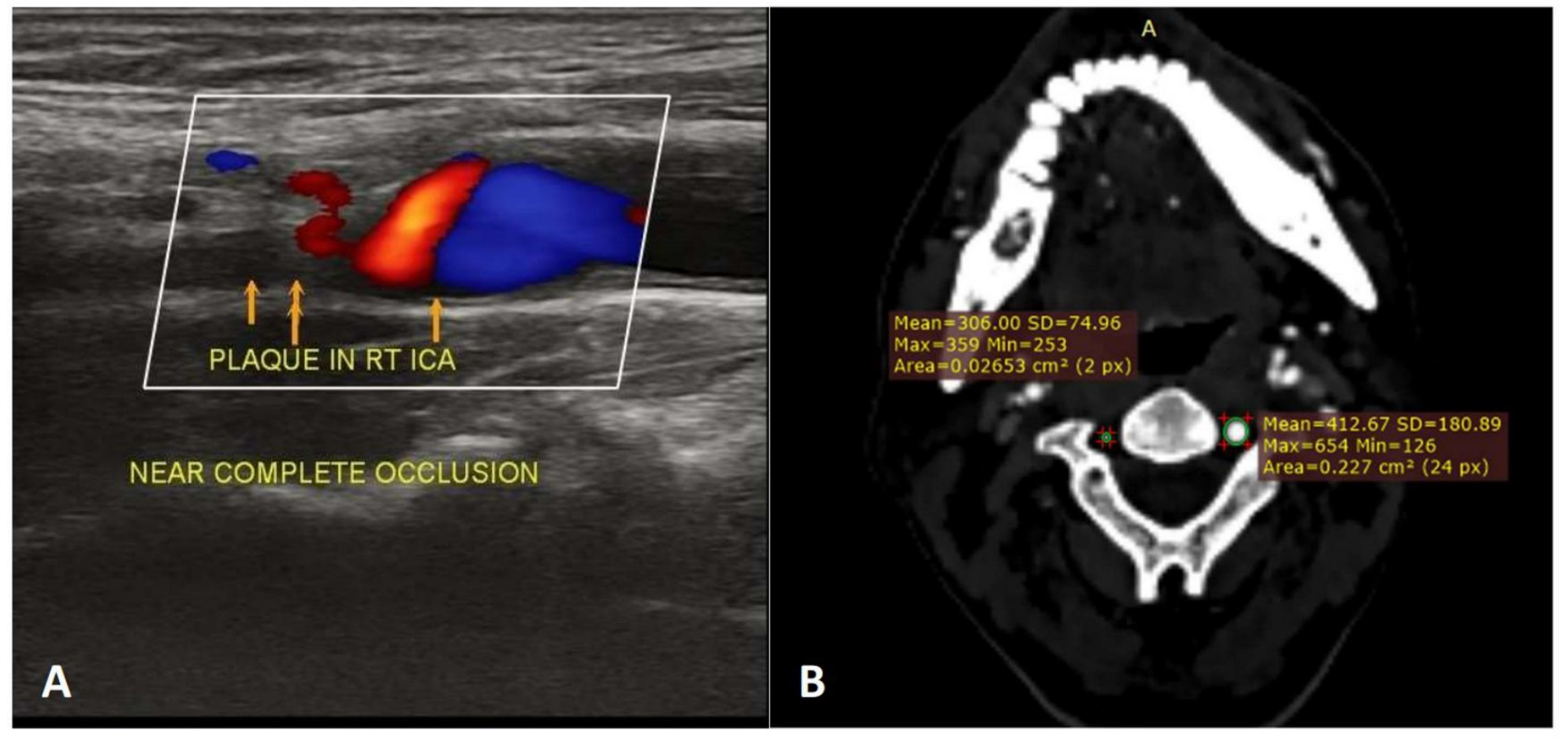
Neuroimaging findings in Case 1. (A) B-mode longitudinal scan CDUS at right carotid level shows plaque in right ICA causing complete occlusion with aliasing on CDUS; and (B) Axial CE-CT of neck vessels showing hypoplastic right VA as compared to normal left side.
